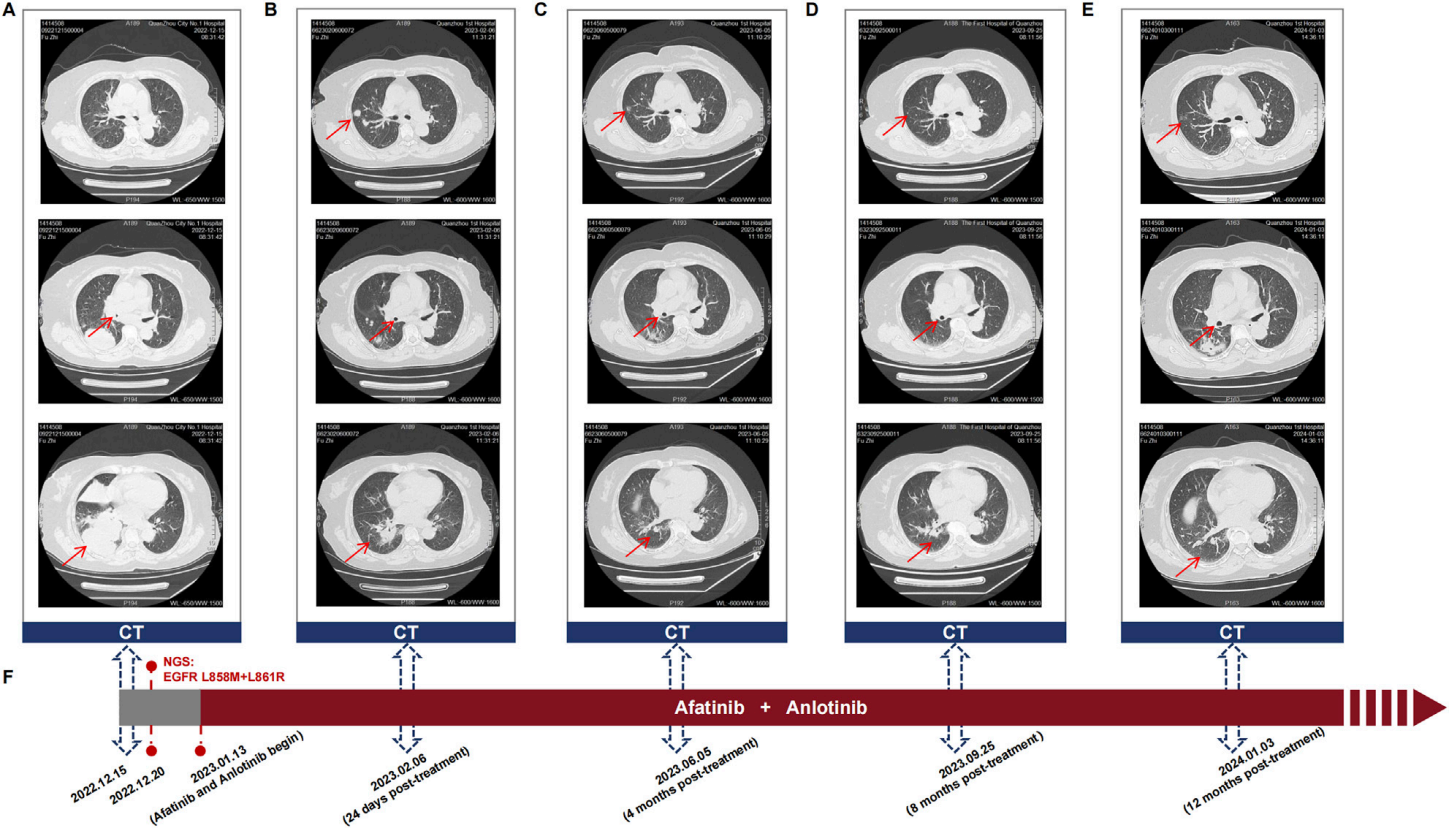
(A–E) Sequential chest CT scans showing the response of the primary lung tumor and metastatic lesions to the combination therapy of afatinib and anlotinib in the patient with *EGFR* L858M/L861R mutations. These scans were taken at (A) diagnosis, (B) 24 days post-treatment, (C) 4 months post-treatment, (D) 8 months post-treatment, and (E) 12 months post-treatment. Upper, middle, and lower CT images indicate the changes in tumor size, bronchial obstruction, and atelectasis, respectively. (F) Timeline of the patient’s treatment course, highlighting key events of diagnosis, mutation detection, initiating afatinib and anlotinib treatment, and follow-up visits.
